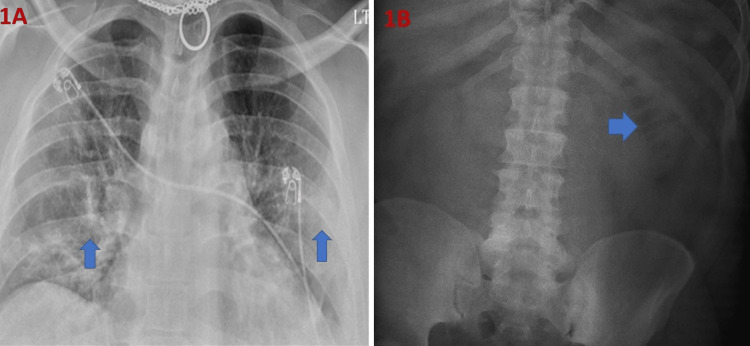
(A): Portable chest X-ray anteroposterior view demonstrated hazy patchy bilateral infiltrates (arrow) (B): Portable X-ray of abdomen supine view demonstrating overall paucity of bowel gas with questionable thickened folds in a partially distended loop of bowel in the left upper abdomen (arrow).
